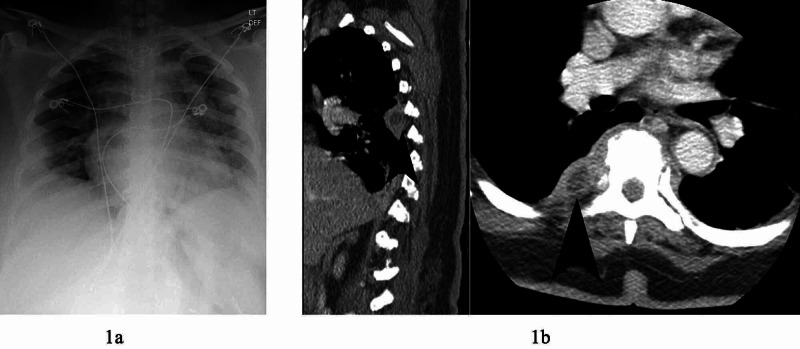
Chest x-ray and CT chest with contrast. 1a: Plain chest x-ray showing decreased lung volume, cardiomegaly, and multiple opacities concerning of coronavirus disease 2019 (COVID-19) infection. 1b: CT chest with contrast demonstrating ring enhancing lesions (arrows) at base of right lung at thoracic vertebrae 5 and 6 in sagittal and axial views.
